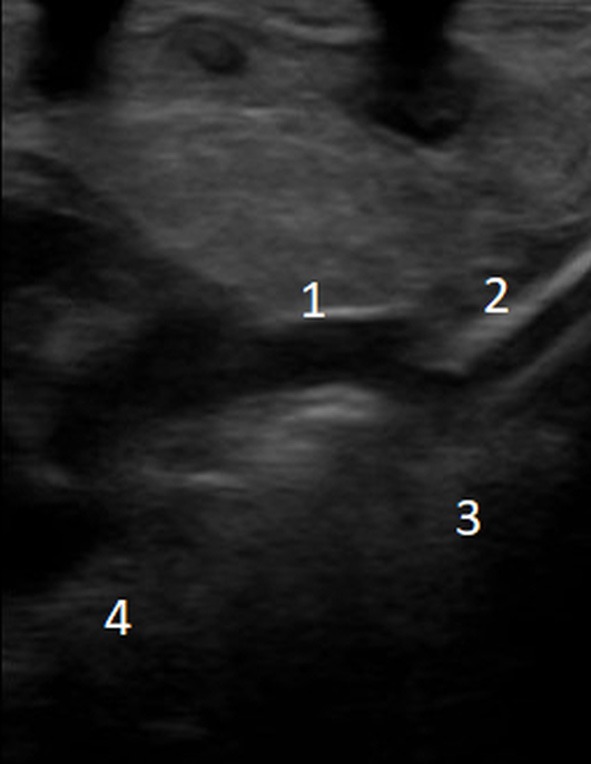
Echocardiographic evaluation of venous cannula position: (1) superior vena cava; (2) venous cannula; (3) azygos vein; (4) right atrium.
